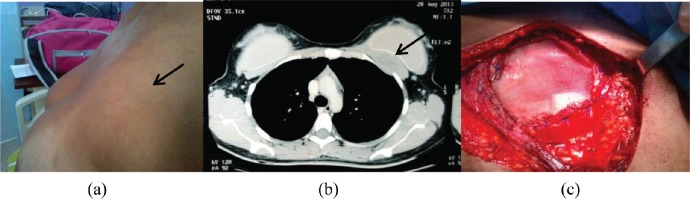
First case. (a) Patient with breast fibromatosis at initial physical exam: asymmetry at the union of the superior quadrants. (b) Computed tomography (CT) of the thorax: area of tumour adjacent to the prosthesis is shown. (c) At the moment of the surgical resection: area of WLE reconstructed with polypropylene mesh.
